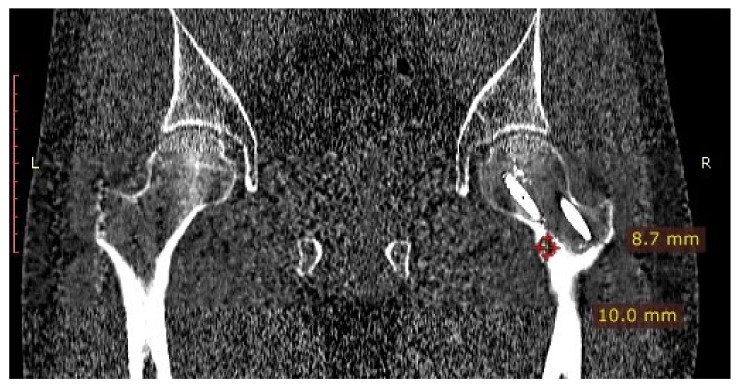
CT scan of the hip joints showing a focus of OO in the right femoral neck.
